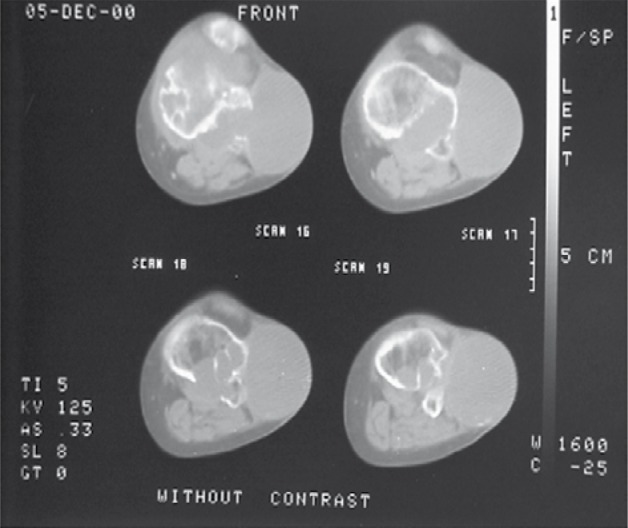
CT Scan Without Contrast in the Same Patient. It shows multiple destructive cystic lesions in the proximal of the tibia and distal of the femur which some of them show extra-osseous extension.
